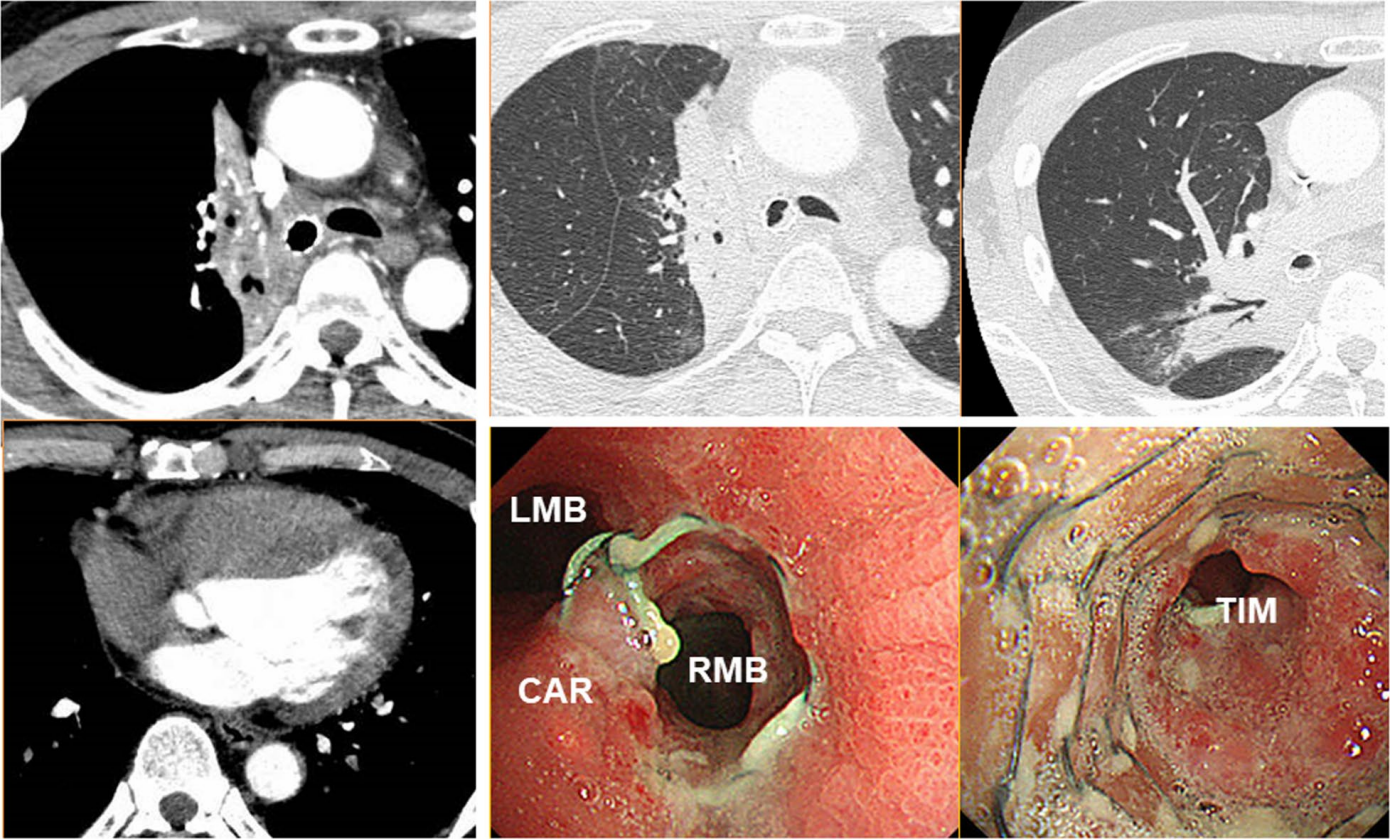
Computed tomography (CT) after bronchial stenting and immuno-chemo-radiotherapy showed dramatic tumor shrinkage and disappearance of pericardial effusion (left), and also showed persistent lung infection (right upper) associated with sent placement (right lower). Microbiological test of the “green–blue” sputum collected by deep coughing and by bronchoscopy revealed bacterial phagocytosis by neutrophils and growth of bacteria that was identified as *Pseudomonas aeruginosa*. CAR, carina; LMB, left main bronchus; RMB, right main bronchus; TIM, truncus intermedius
